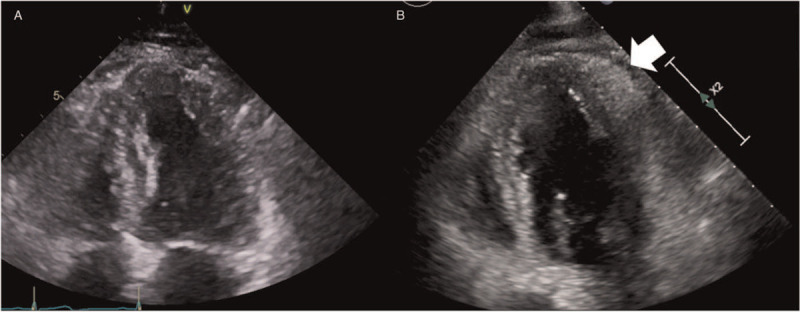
TTE 1 year ago was a normal study (A). TTE after PCI showed focal areas of myocardial thickening and associated hypokinesis with an adherent, mobile echo density attached to the inferolateral myocardium (B). TTE = trans thoracic echocardiogram, PCI = percutaneous coronary intervention.
